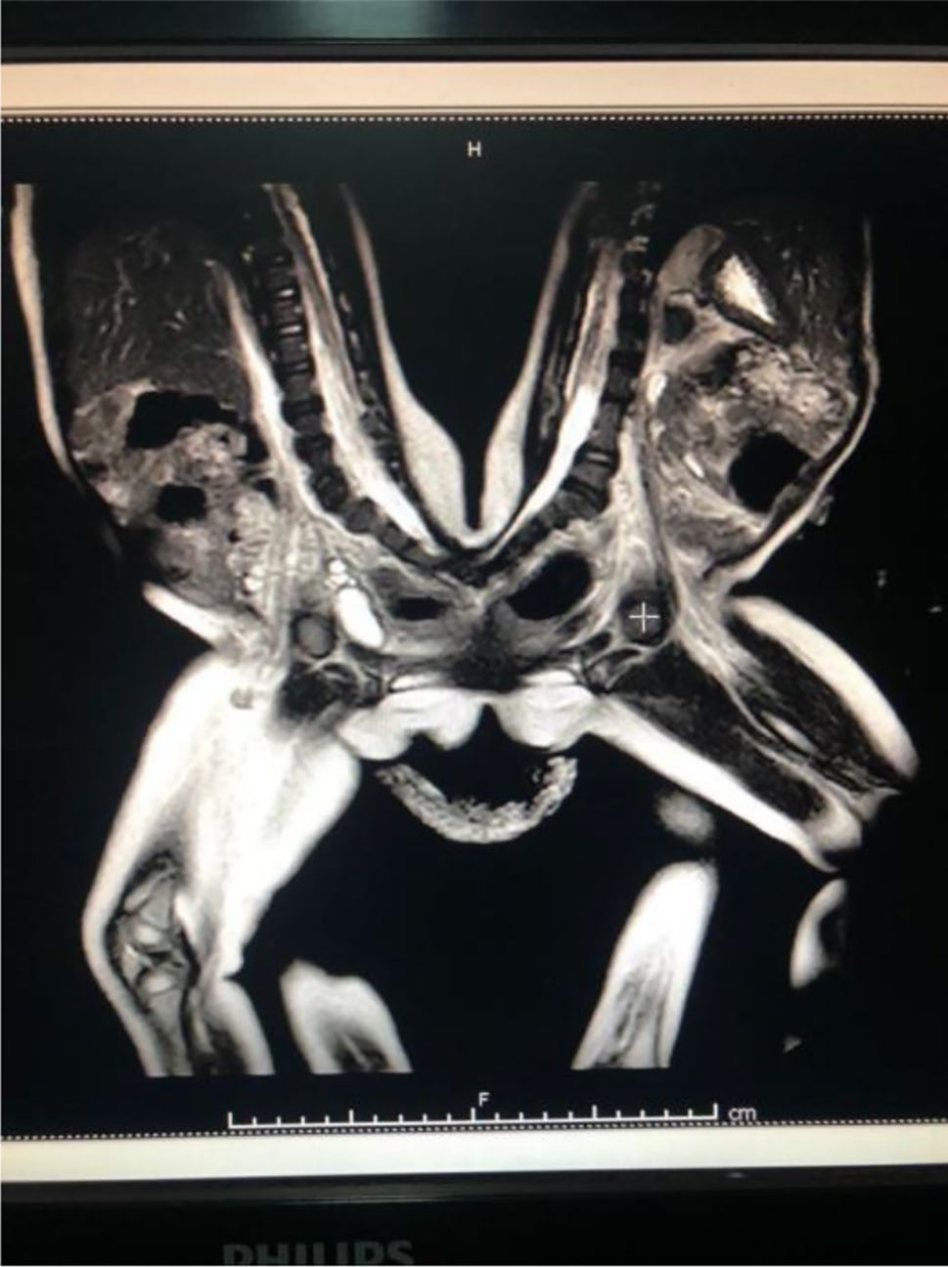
MRI of the pygopagus twins
